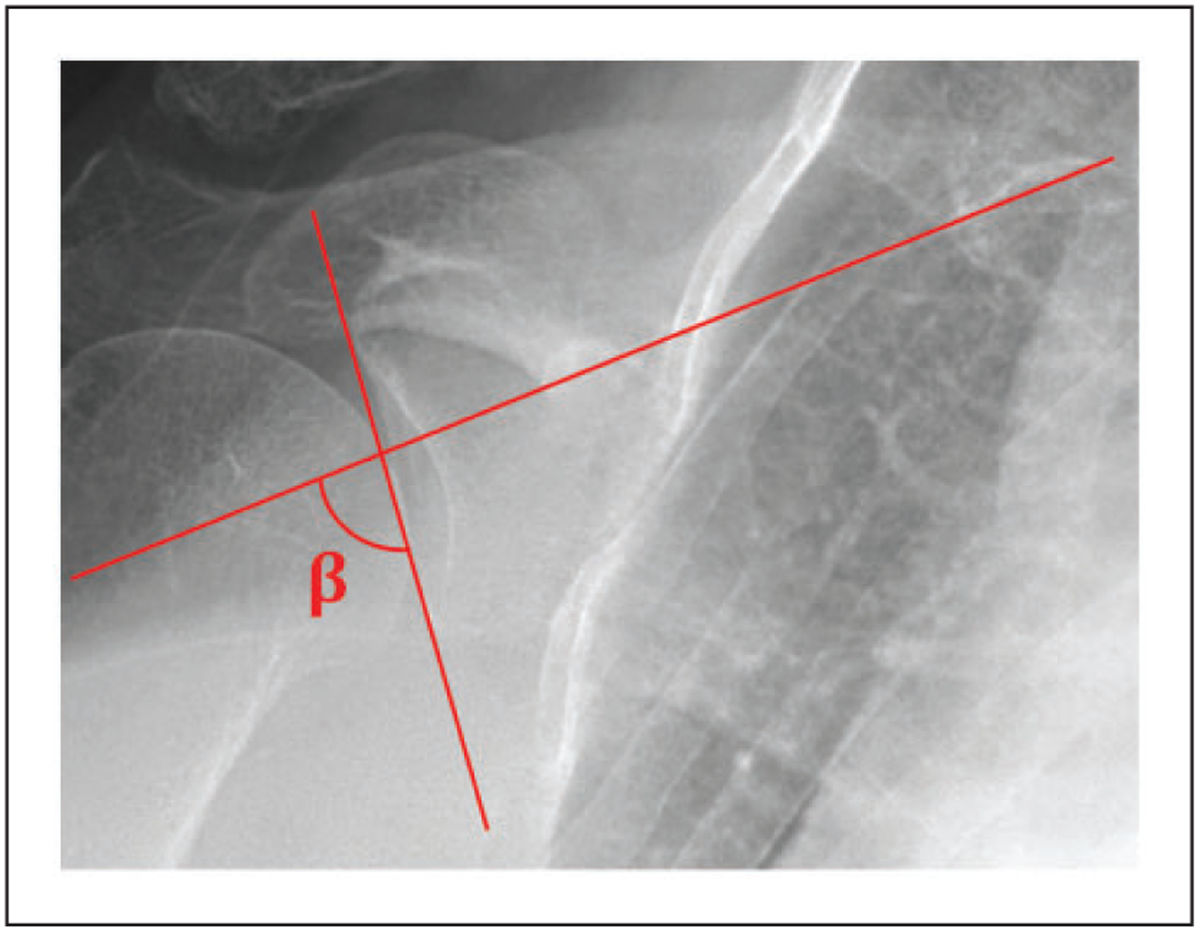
Measurement of the 2D *β* angle on a digitally reconstructed radiograph as the angle between the supraspinatus fossa (horizontal) and a line between the inferior and superior glenoid poles (vertical).
